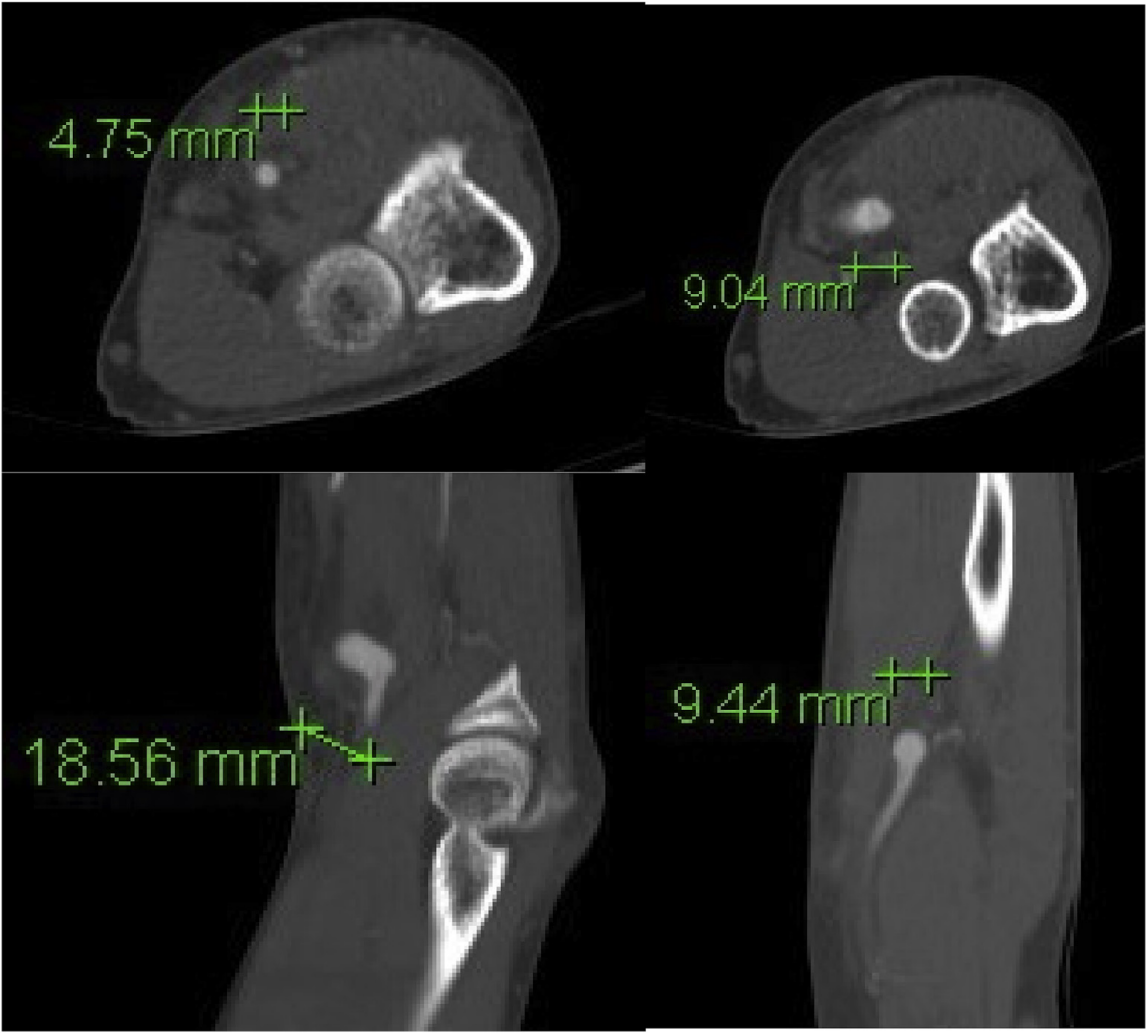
Axial CT angiogram images of the left upper extremity with a normal caliber brachial artery and 9 mm aneurysm of the brachial artery just distal to this at the level of the elbow (top row). Coronal and sagittal CT angiogram of the brachial artery aneurysm (bottom row).
